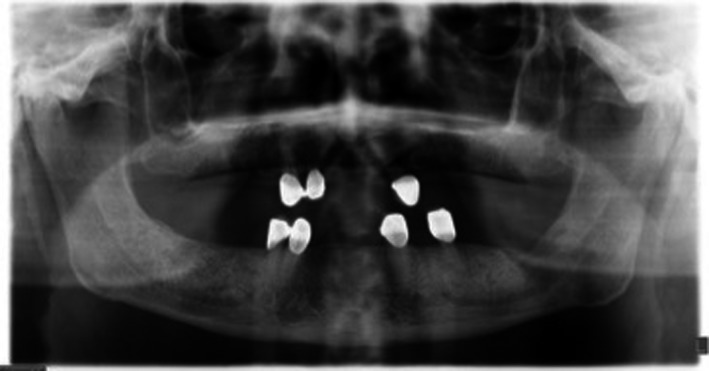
Panoramic Radiography was taken after 3 mo and Shows no Calcification in the Lesion
